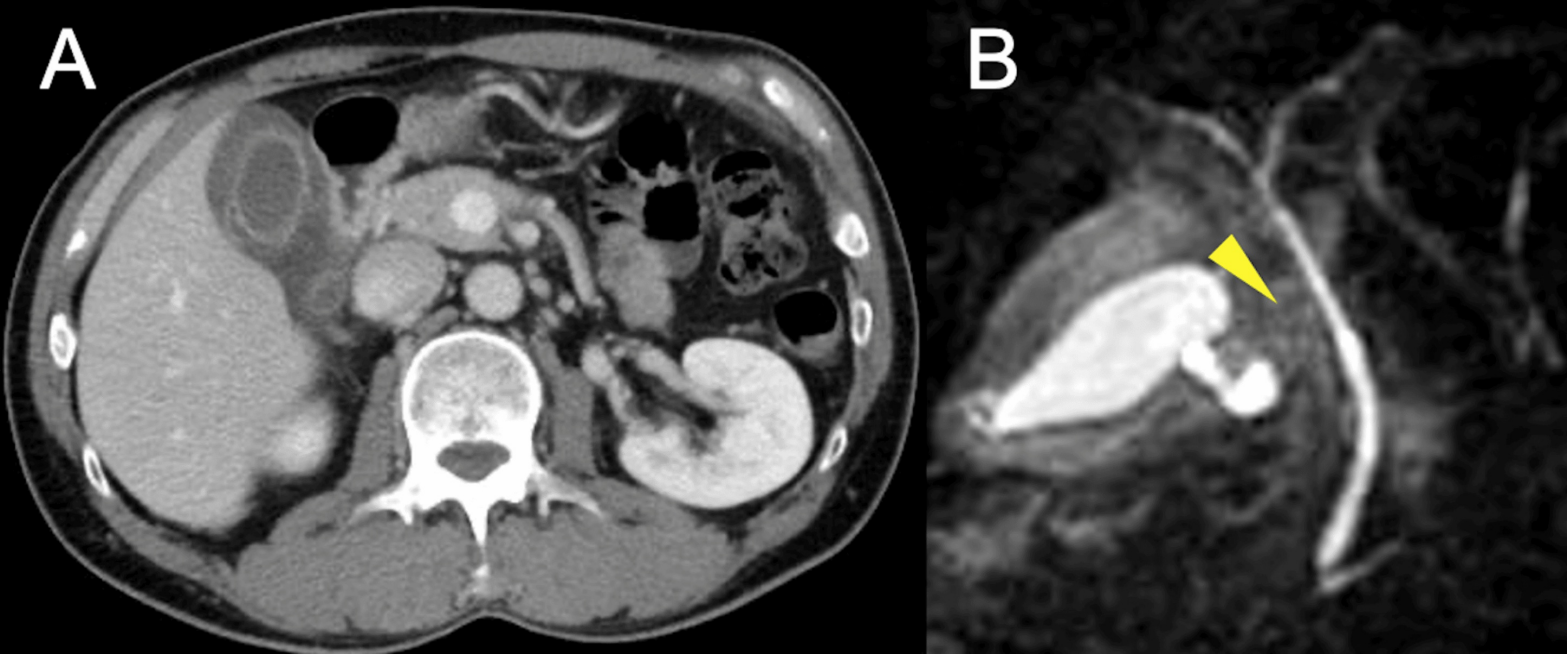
Radiological findings of gallbladder volvulus in Case 2 (A) CT image shows significant thickening and edema of the gallbladder wall. (B) MRCP image reveals a torsion-like defect at the level of the gallbladder neck.
